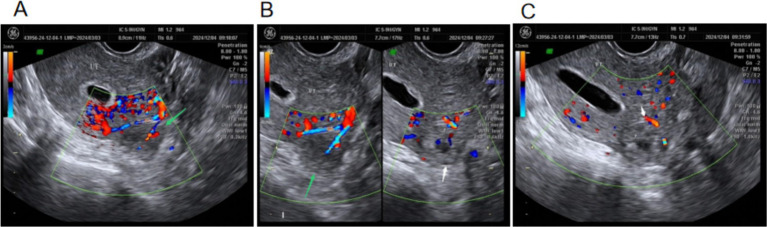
Ultrasound images on day 17 of conservative treatment. (A) Thrombus formation within the UAP. (B) Coexistence of UAP and left interstitial tubal pregnancy. (C) Reduced blood flow within the left interstitial pregnancy lesion.
